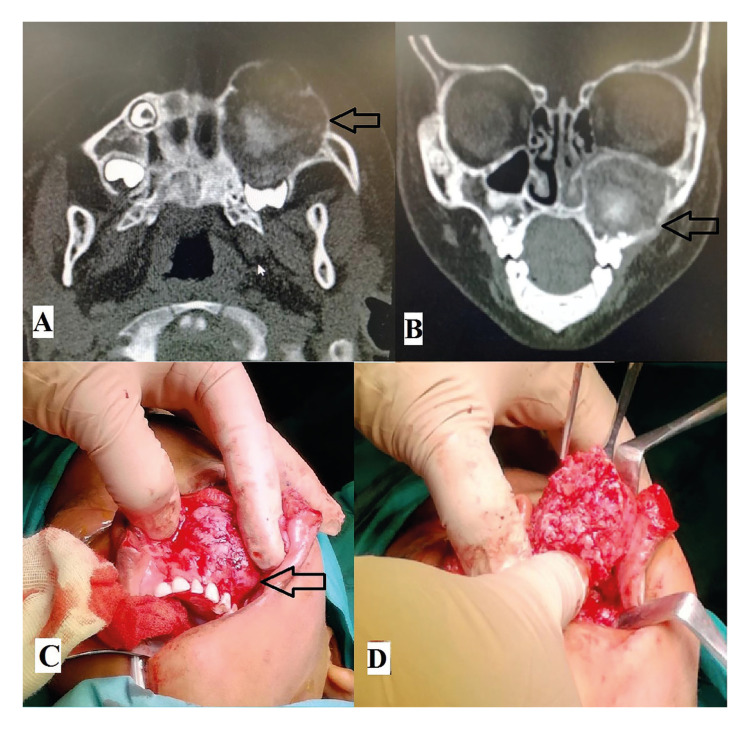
A: Axial CT showing mixed density JTOF lesion with central radio-opacity. B: Coronal CT of same patient showing extension of tumour into maxillary antrum and nasal cavity. C: Intra-operative exposure of neoplasm via Weber-Ferguson incision. D: Curettage of the lesion which was un-encapsulated but well demarcated.
